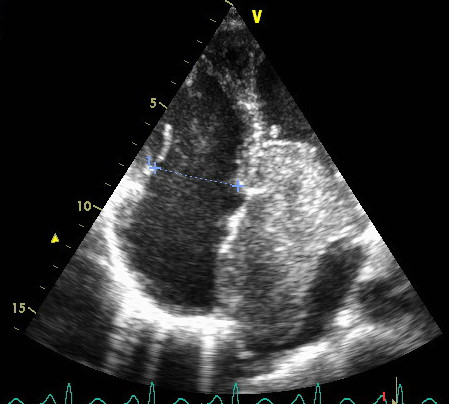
Transthoracic echocardiography 4 cavity view shows a giant left atrial myxoma fills almost the entire left ventricular at diastole.
